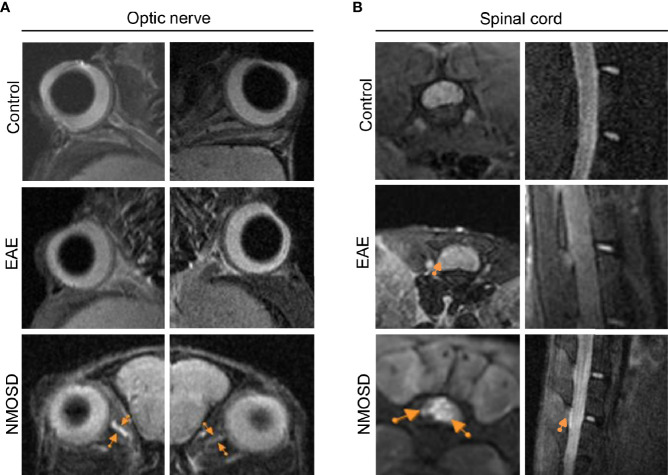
MRI presentation in the NMOSD model. (A) Both sides of the optic nerve in the NMOSD model showed increased intensity (arrows) on T2 FLAIR imaging, especially on the right side; (B) Increased intensity (arrows) was found in both transverse and longitudinal images of the spinal cord.
